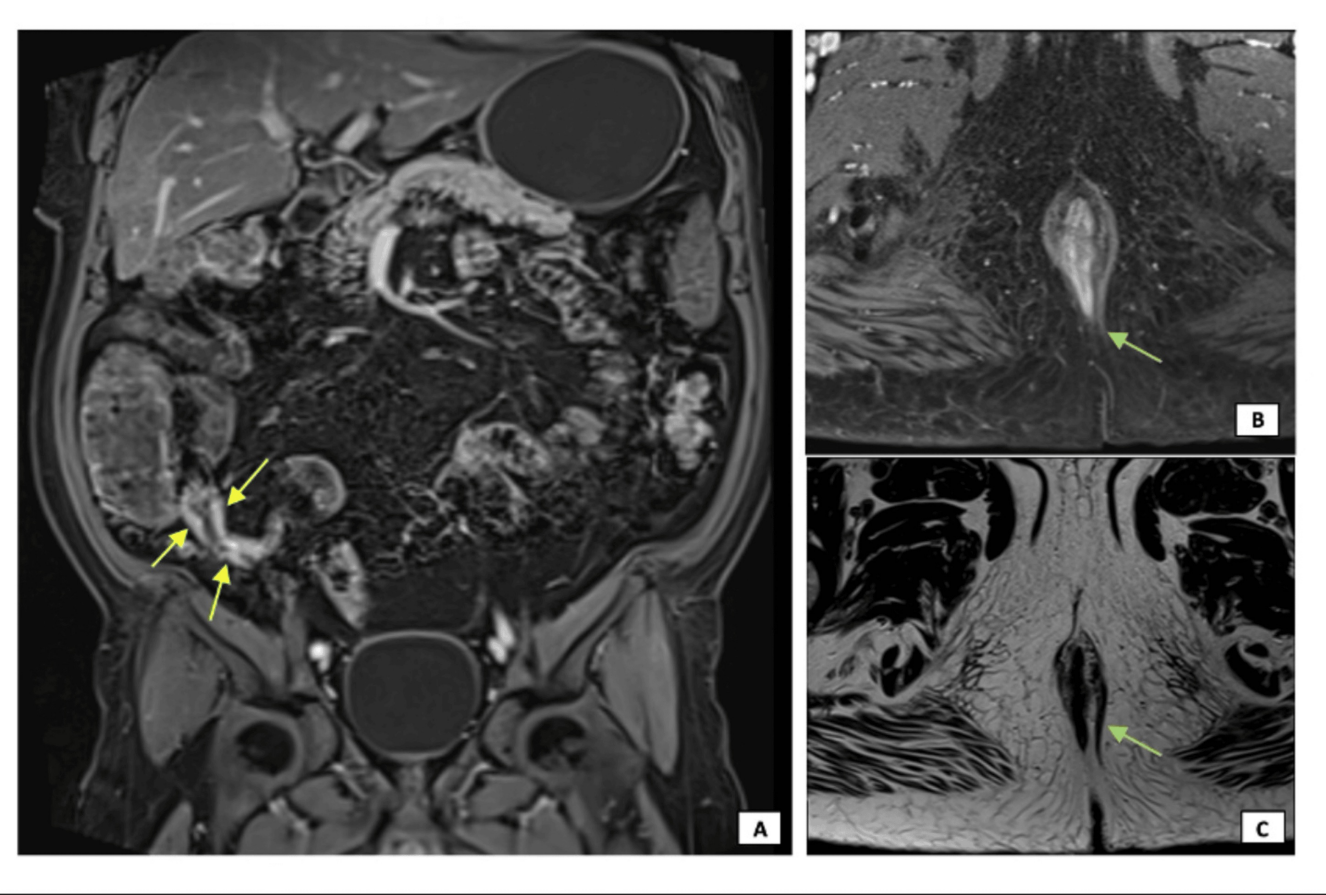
MR imaging of the pelvis and enterography demonstrating terminal ileum wall thickening, with contrast enhancement (A, yellow arrows), and an anal fistula with intersphincteric fistulous tract (B and C, green arrows), both findings suggestive of CD MR: magnetic resonance; CD: Crohn’s disease
